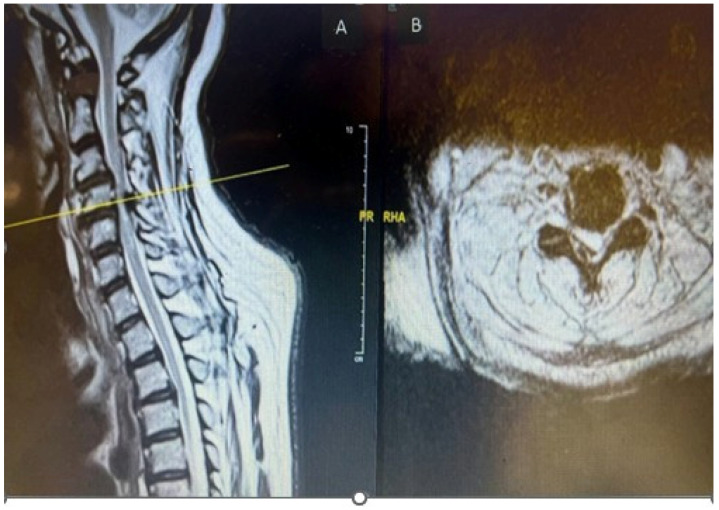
Preoperative MRI cervical spine T2-weighted sagittal (A) and axial (B) showing cord compression posteriorly with cord signal change at cervical 4 level.
